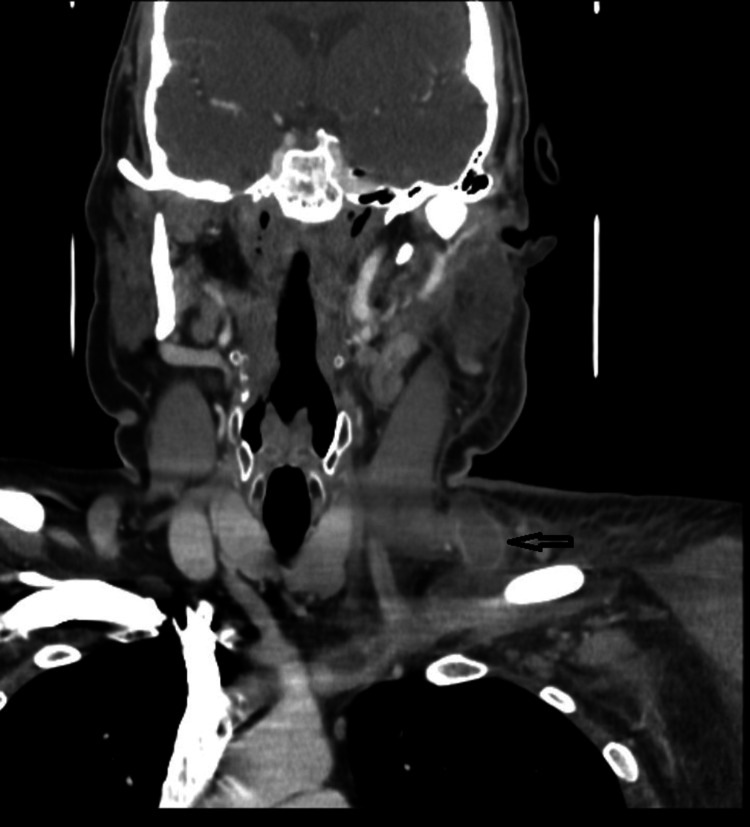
CT scan of the neck coronal section showing thrombus in the left external jugular vein and swelling of left side soft tissue
